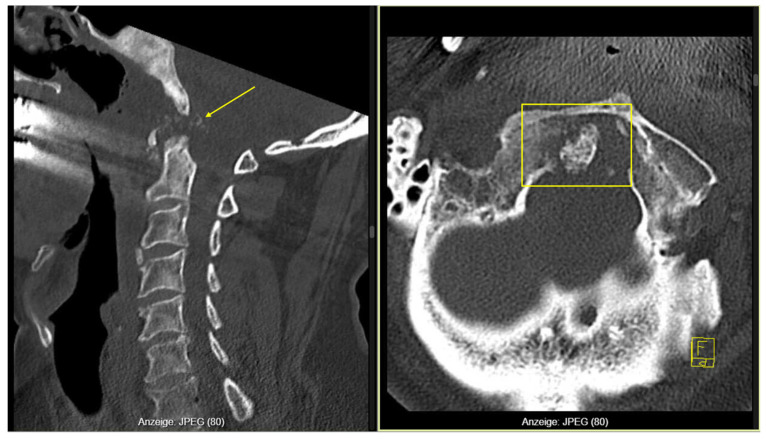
Cervical CT showing C1 osteolysis with Pannus (arrow) and atlantoaxial subluxation (frame).
